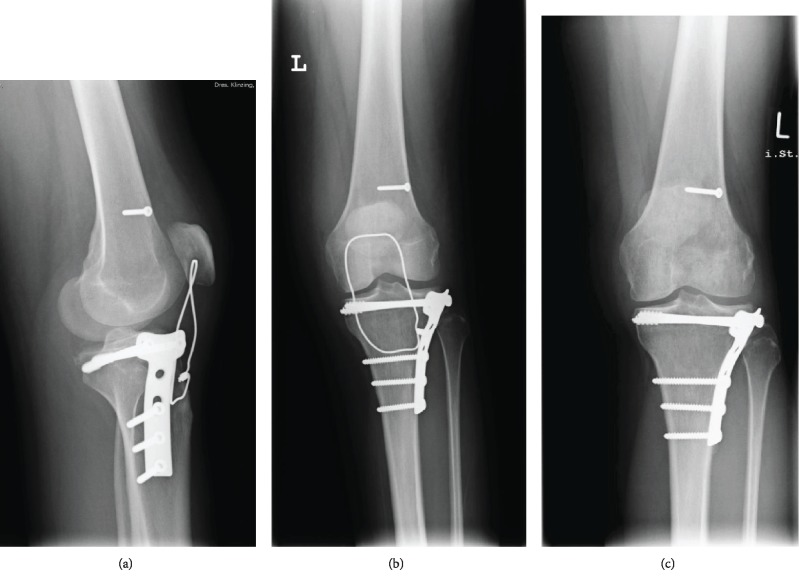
Radiographic result after the operation (a, b) and after the removal of the McLaughlin wire (c).
